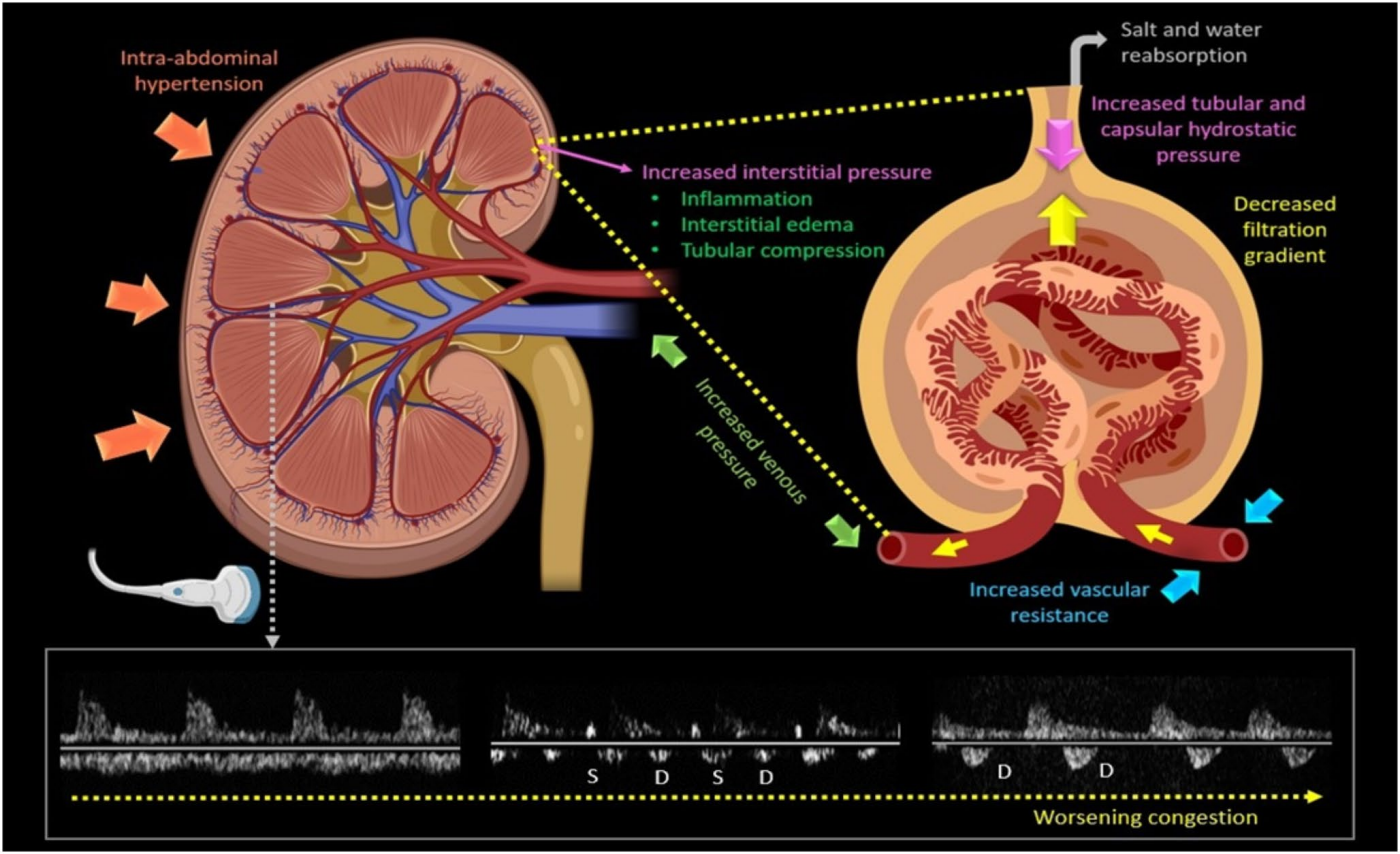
Pathophysiology of renal congestion and corresponding intrarenal Doppler patterns. As congestion worsens, renal venous flow (bottom, left to right) transitions from continuous to discontinuous. Initially, it becomes biphasic with distinct systolic (S) and diastolic (D) waves, and eventually, it becomes monophasic, with blood flow occurring only during diastole (D). *Figure made using Biorender®*
